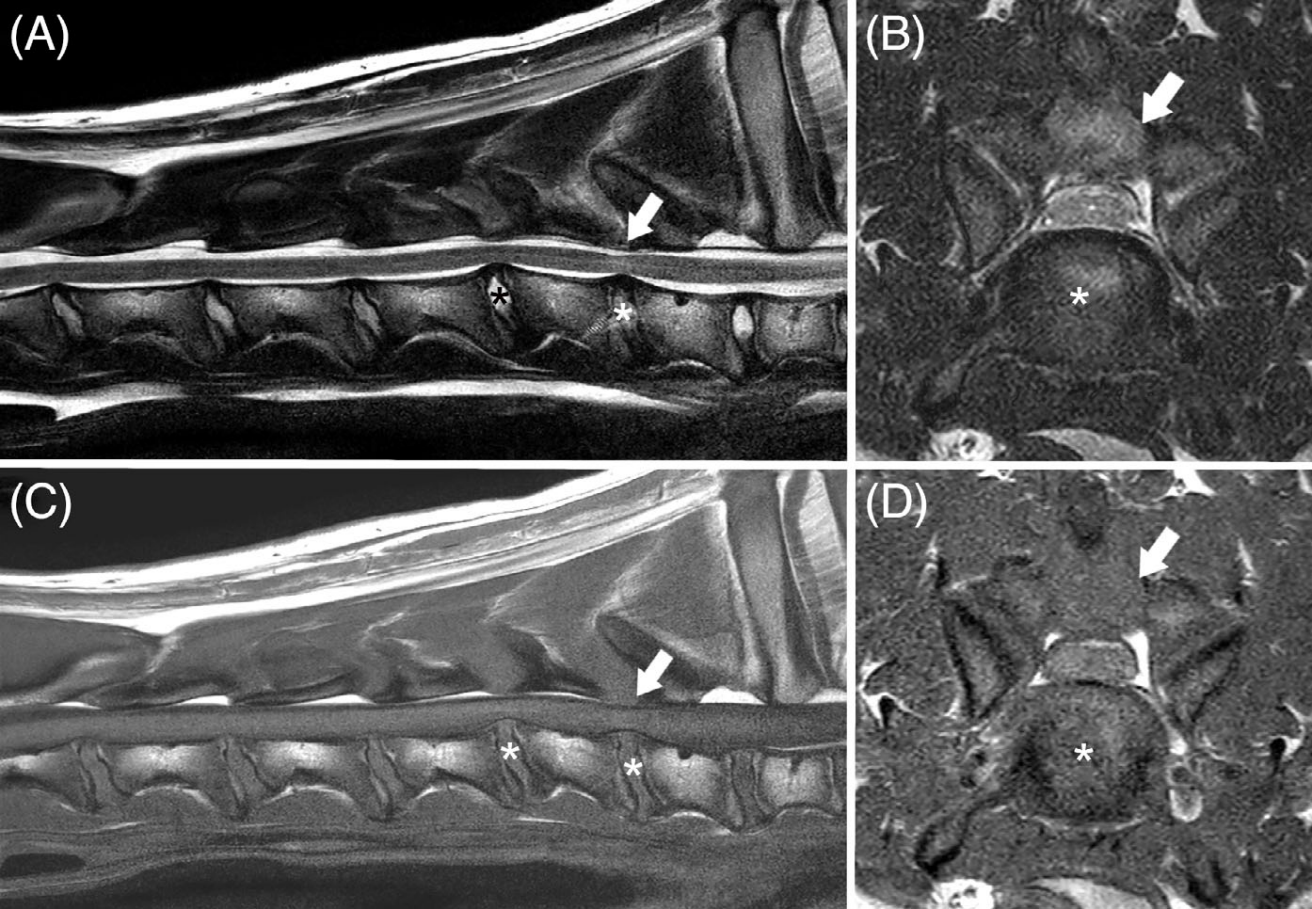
T2‐weighted sagittal (A) and transverse (B) and T1‐weighted sagittal (C) and transverse (D) magnetic resonance imaging of a 6‐month‐old Great Dane with cervical spondylomyelopathy because of intervertebral disk protrusion (asterisks) at C5‐C6 and C6‐C7 and dorsal soft tissue proliferation (arrow) with mild C6 dorsal lamina thickening at C6‐C7
